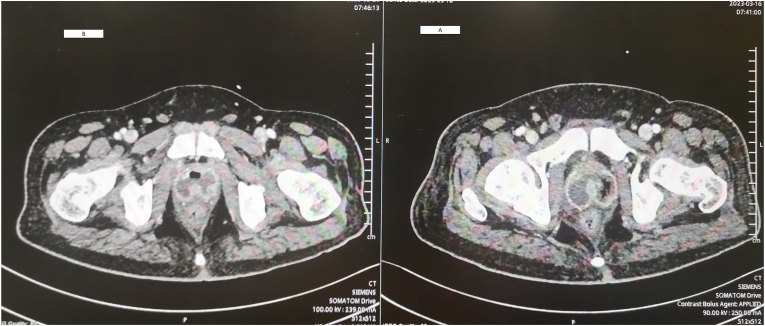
Repeated CT abdomen and pelvis with contrast showed the difference between a. After the first and b. After the second resection.
